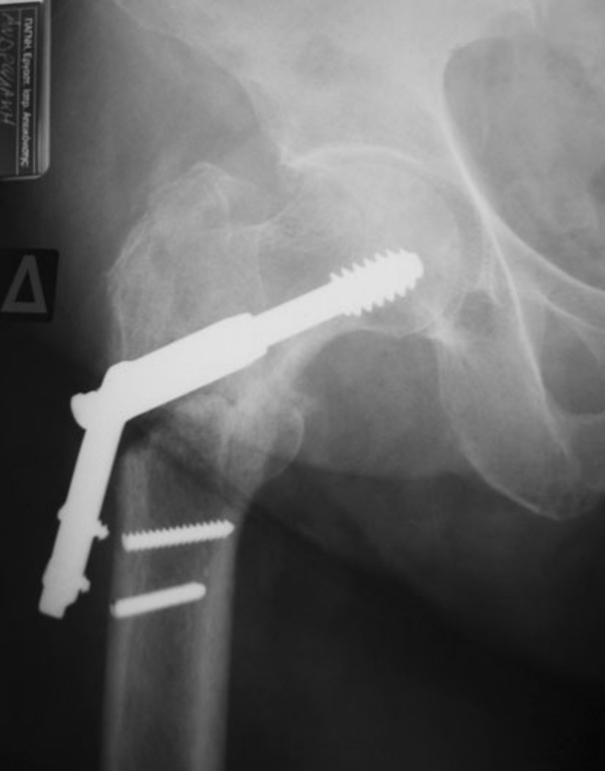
X-ray showing the barrel-plate pulled off the femur as a result of a fall 4 months postoperatively
